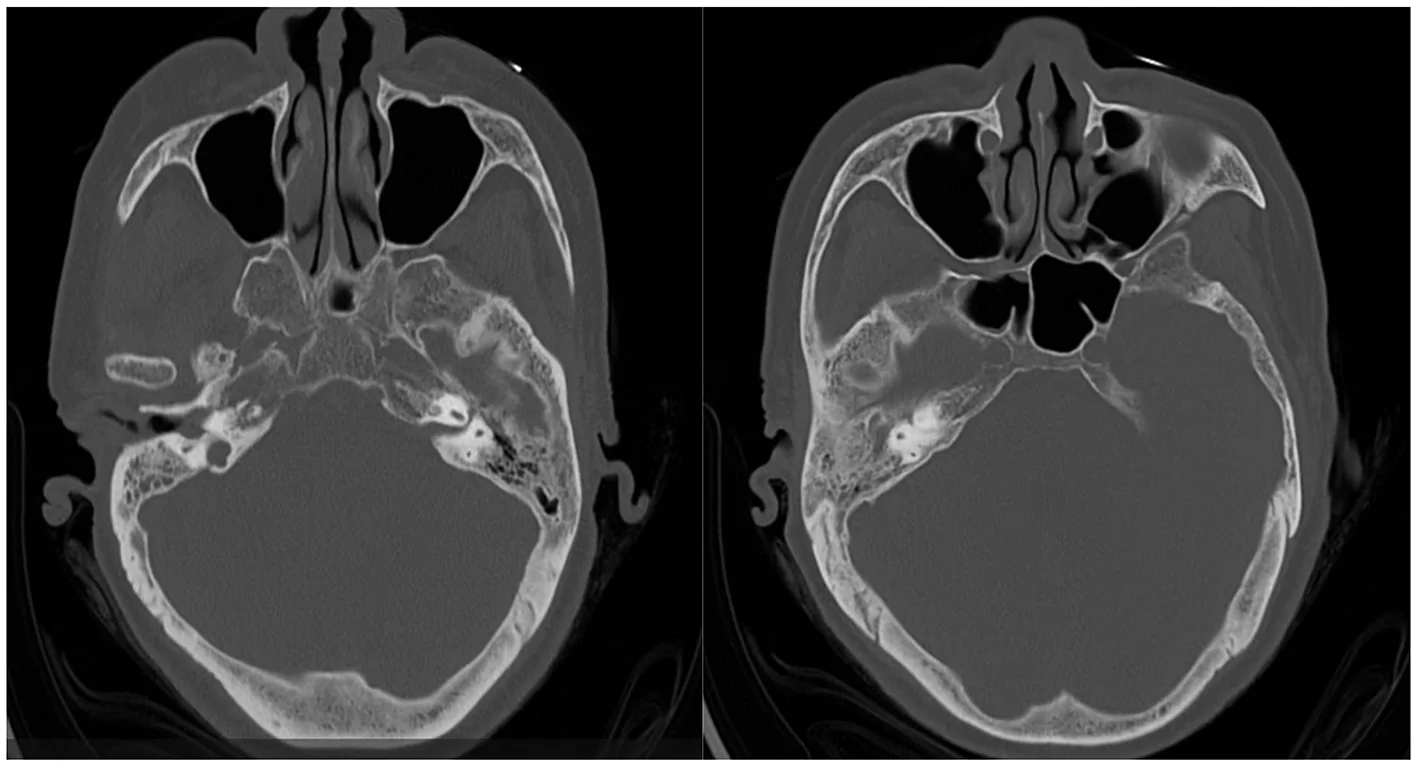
CT of the middle ear on the 6th day of hospitalization. Poor aeration of the right mastoid; areas of increased density are visible within the tympanic cavity and tympanic sinus. There is no obvious compression, displacement, erosion, or destruction of the ossicles; there is no enlargement of the tympanic cavity or tympanic sinus. No abnormal changes were observed in the inner ear structures, and no narrowing or dilation was noted in the external auditory canal.
